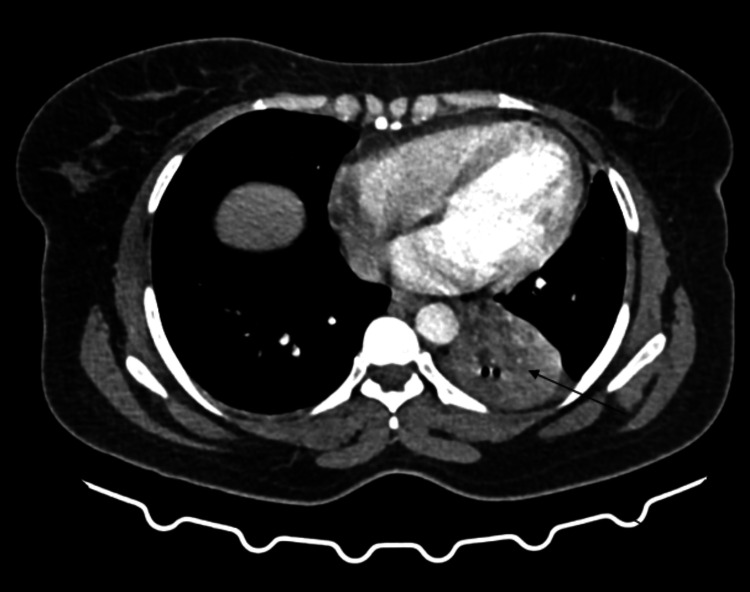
The corresponding contrast-enhanced computed tomography (CECT) thorax axial section demonstrates a fairly defined, homogeneously enhancing soft tissue lesion in the left lower lobe, accompanied by dense collapse-consolidatory changes and a few air bronchograms (black arrow).
